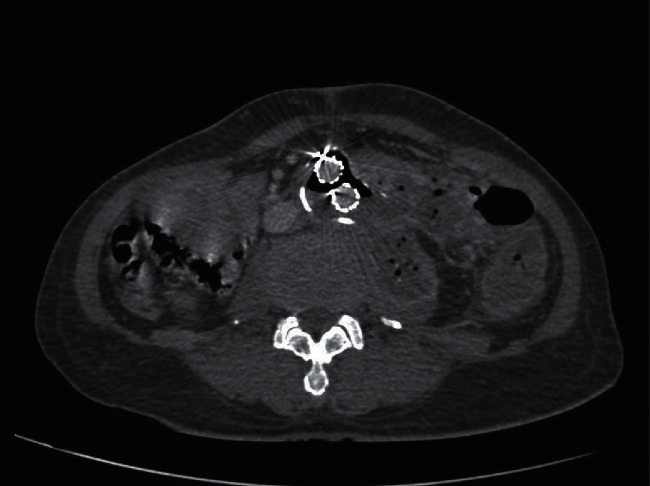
CTA revealed air in the aneurysm sac and left psoas abscess.
